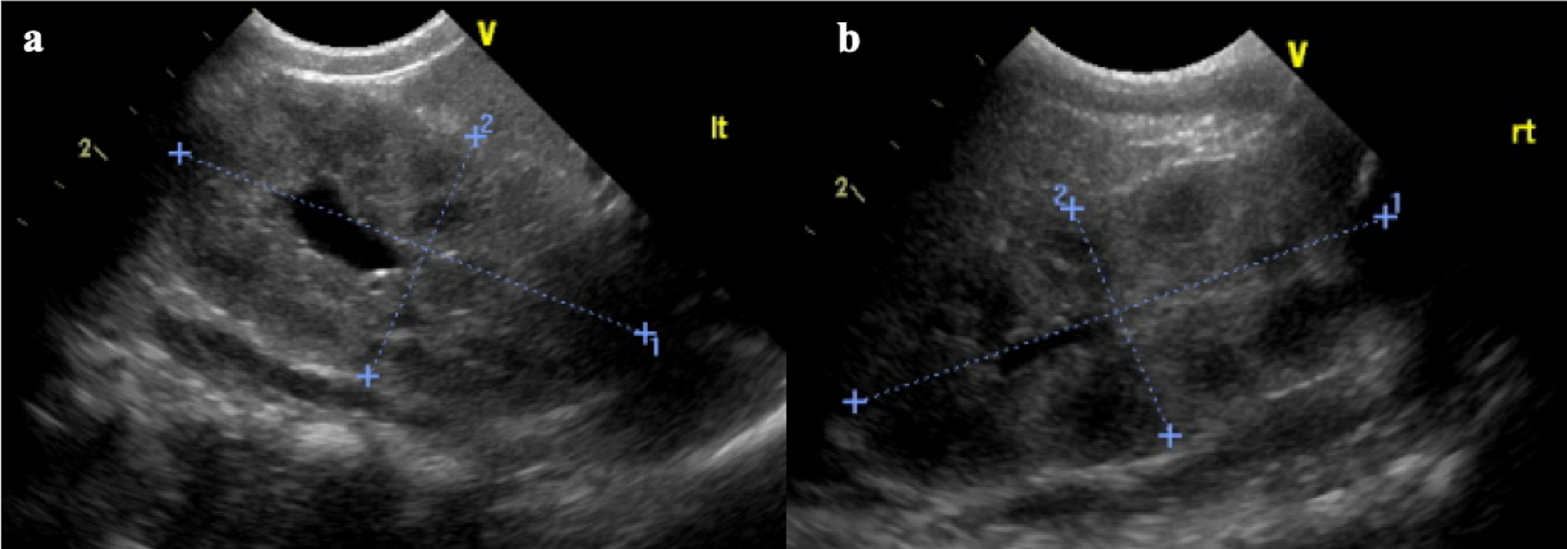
Ultrasound imaging of hydronephrosis (a) Left kidney has renal pelvis dilation, showing grade 1 hydronephrosis. (b) Right kidney without renal pelvis dilation.
